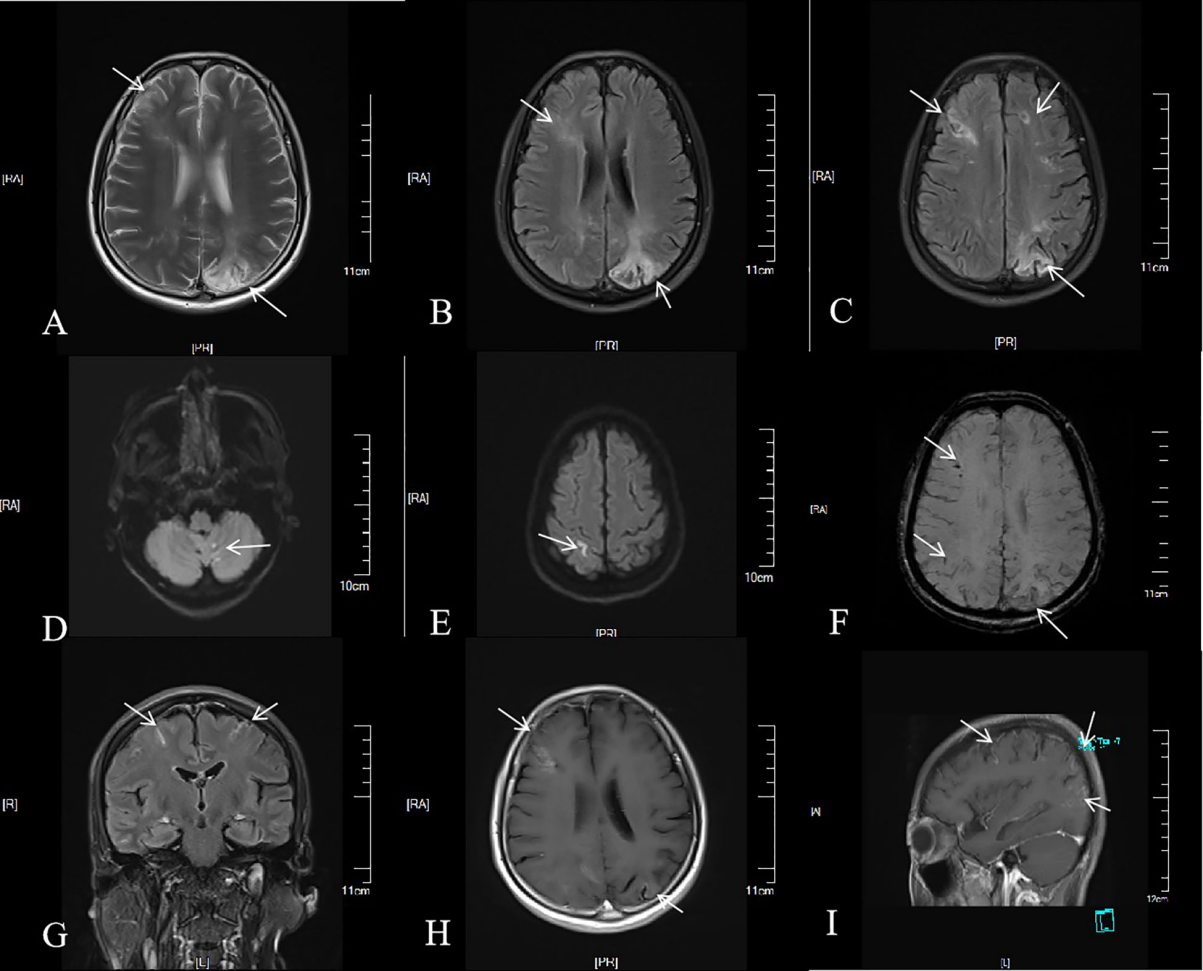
Brain MRI of the patient. Multiple patchy long T2 signals were seen in the cortex, subcortex and white matter of the right frontal lobe of the left occipital lobe (A). On FLAIR sequence, multiple punctate and patchy high signals were seen in the cortex, subcortex and white matter of the bilateral frontal, parietal and occipital lobes (B, C). Cerebellar vermis punctate, left parietal lobe linear high signal on DWI; b = 1,000 (D, E). Multiple low signals were found in the left frontal lobe, parietal lobe and right occipital lobe on SWI (F). Linear enhancement signals were seen in the pia mater, left occipital lobe, right frontal lobe, bilateral parietal lobe, cortex and subcortex (G, H), and circular enhancement signals were seen in the sagittal subcortex and white matter (I).
